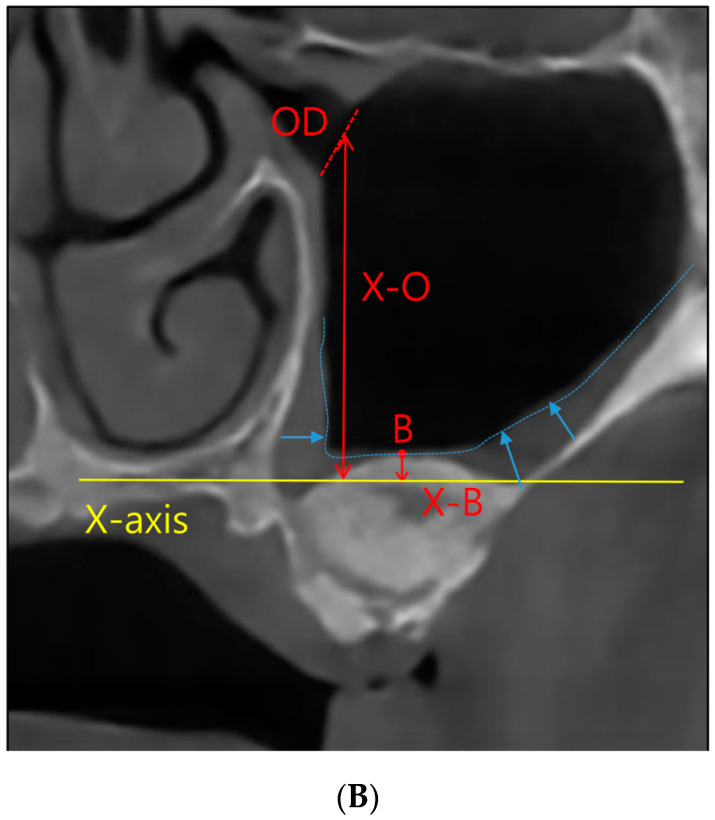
Coronal view of cone-beam computed tomographic images of the maxillary sinus. (A) Preoperative view. X-axis, nose floor in coronal view; OD, maxillary sinus ostium diameter; F, the floor of the maxillary sinus; X-F, distance between the X-axis and floor of the maxillary sinus; X-O, distance between the X-axis and maxillary sinus ostium; MSMT, maxillary sinus mucosal thickness; X-F + X-O, maxillary sinus ostium height/distance between the maxillary sinus ostium and sinus floor. (B) Postoperative view of sinus augmentation and bone graft. X-B, distance between the X-axis and sinus augmentation, X-F + X-B, increase in alveolar bone height in sinus augmentation.
